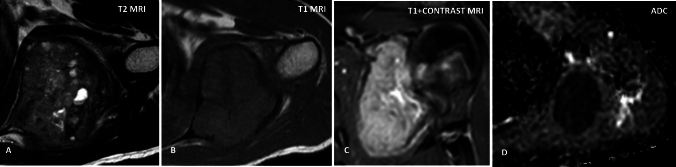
Typical MRI features of chondroblastoma. A Axial T2W image demonstrates T2 hypointensity with few interspersed T2 hyperintense foci. B Axial T1W image shows a T1 intermediate signal intensity lesion with T1 hypointense rim. Coronal T1 + C (C) depicts post-contrast enhancement. In apparent diffusion coefficient map (D), the lesion shows significant restricted diffusion (seen as dark signal)
